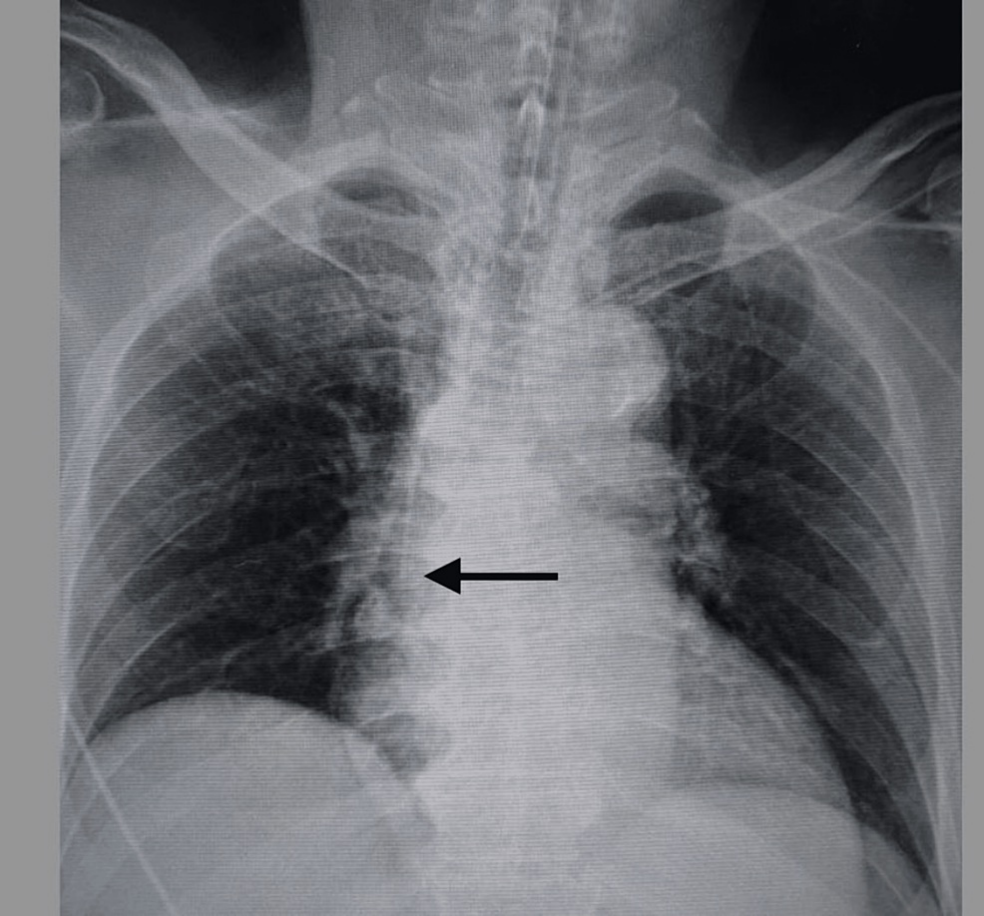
Chest X-ray after insertion of the Power PICC The black arrow was the catheter tip of the Power PICC guided by the Sherlock 3CG system in Case 1.
